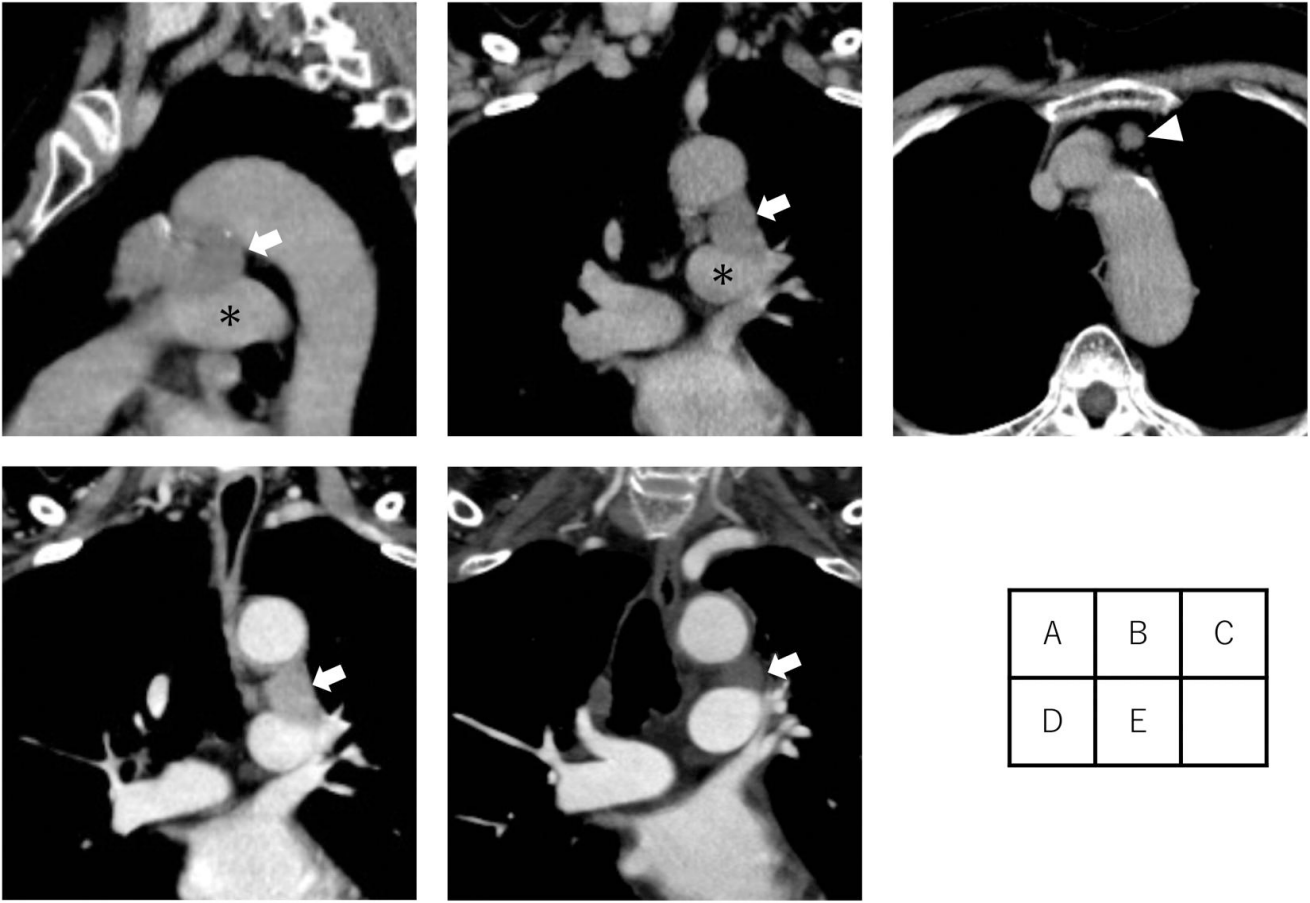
Preoperative CT Findings. (A, B) Anterior mediastinum mass (arrow), which is suspected to have invaded the left main pulmonary artery (asterisk). (C) Enlarged mediastinal lymph nodes (arrowhead). (D) No change in tumour size after chemoradiotherapy (arrow). (E) Tumour reduction after immune checkpoint inhibitor therapy (arrow). Abbreviation: CT, computed tomography.
